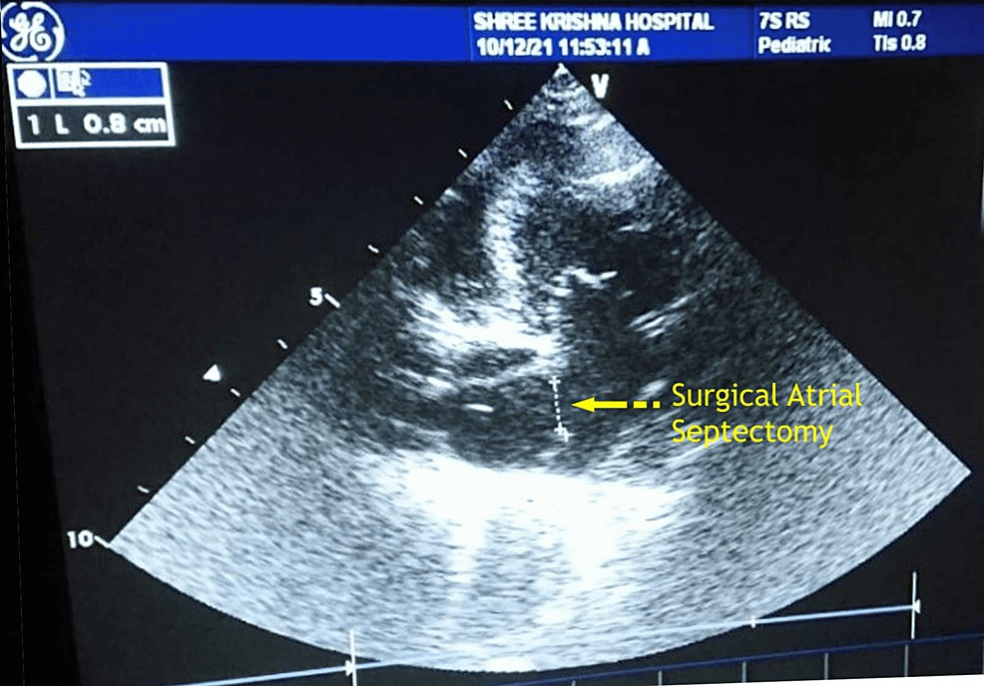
Confirmation of the atrial septectomy procedure with the 2D echocardiography in case 1. 2D: two-dimensional. Image credits: Dr. Vishal V. Bhende.
